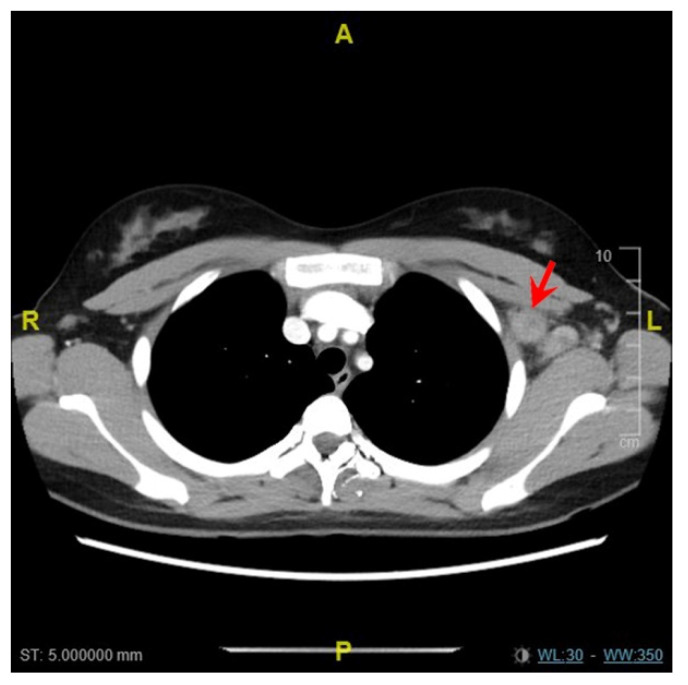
Computed tomography scan of patient A demonstrating enlarged left subpectoral and axillary lymph nodes (red arrow).
